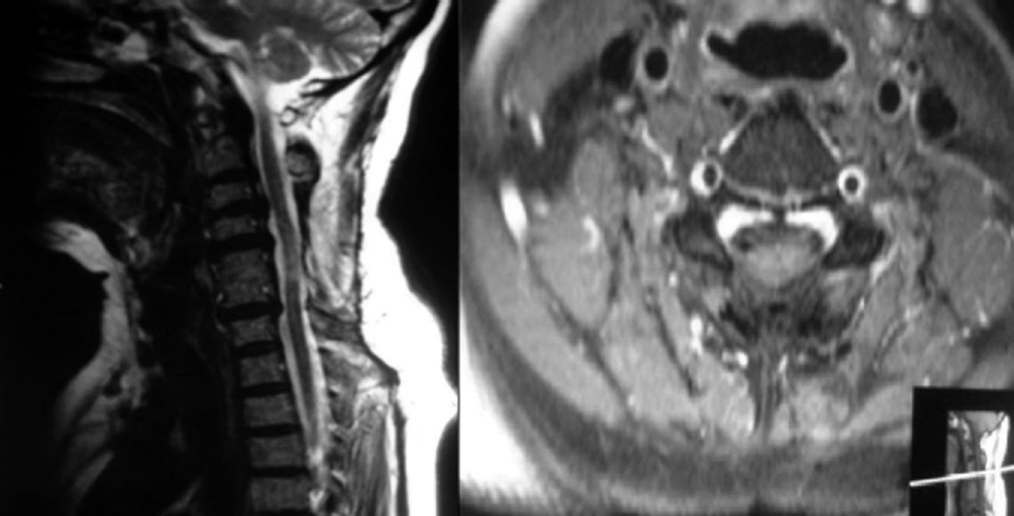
Two-year postoperative sagittal and axial MRI images after tumor excision
